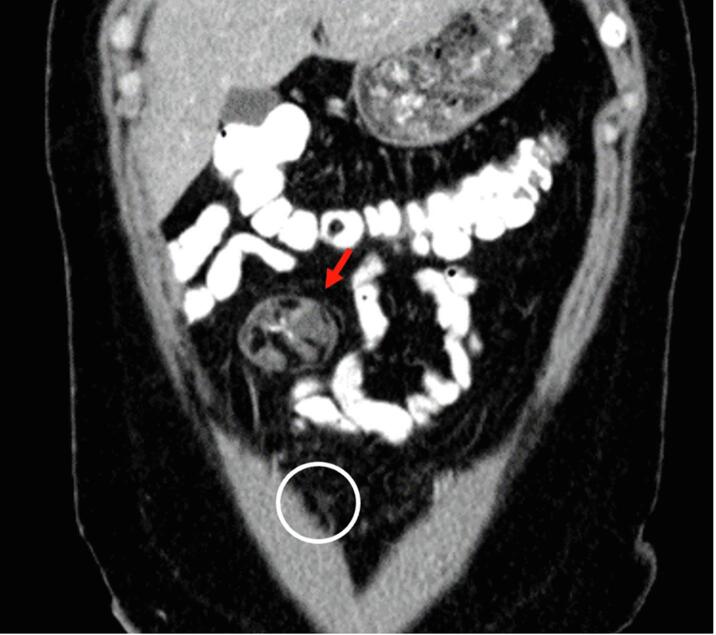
Ten months after surgical resection of immature teratoma, and seven months after chemotherapy completion the restaging CT demonstrates a 5 cm mass (red arrow), predominantly fatty with single calcification, with similar appearance to mature teratoma demonstrated in the CT performed at presentation. There is also trace peritoneal stranding (white circle), which is difficult to distinguish from benign process such as non-specific scarring, trace fluid, early/subtle malignant involvement of peritoneum, or gliomatosis peritonei. (For interpretation of the references to colour in this figure legend, the reader is referred to the web version of this article.)
